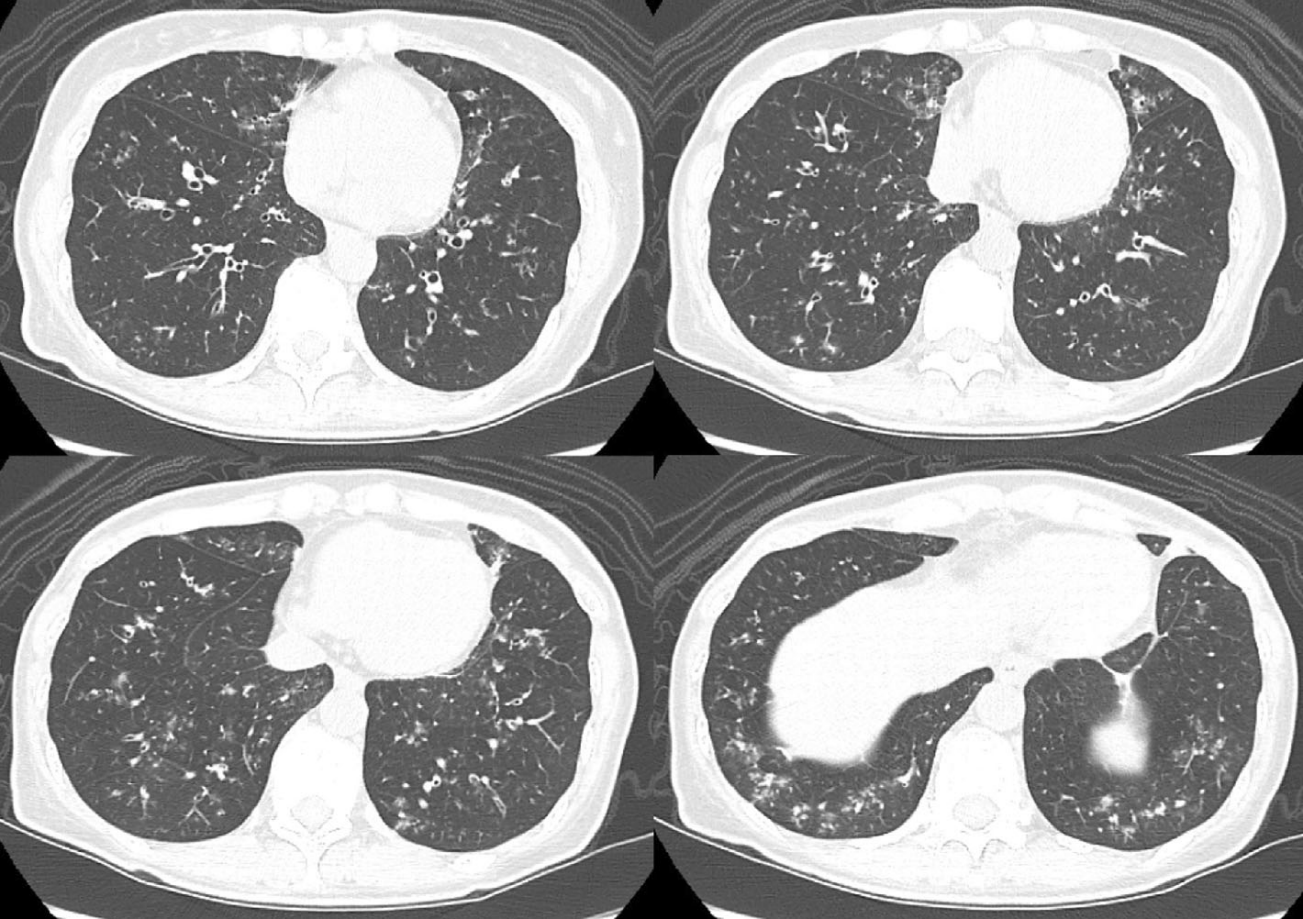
Chest computed tomography (CT) taken after symptom onset, revealing bronchiolitis.
